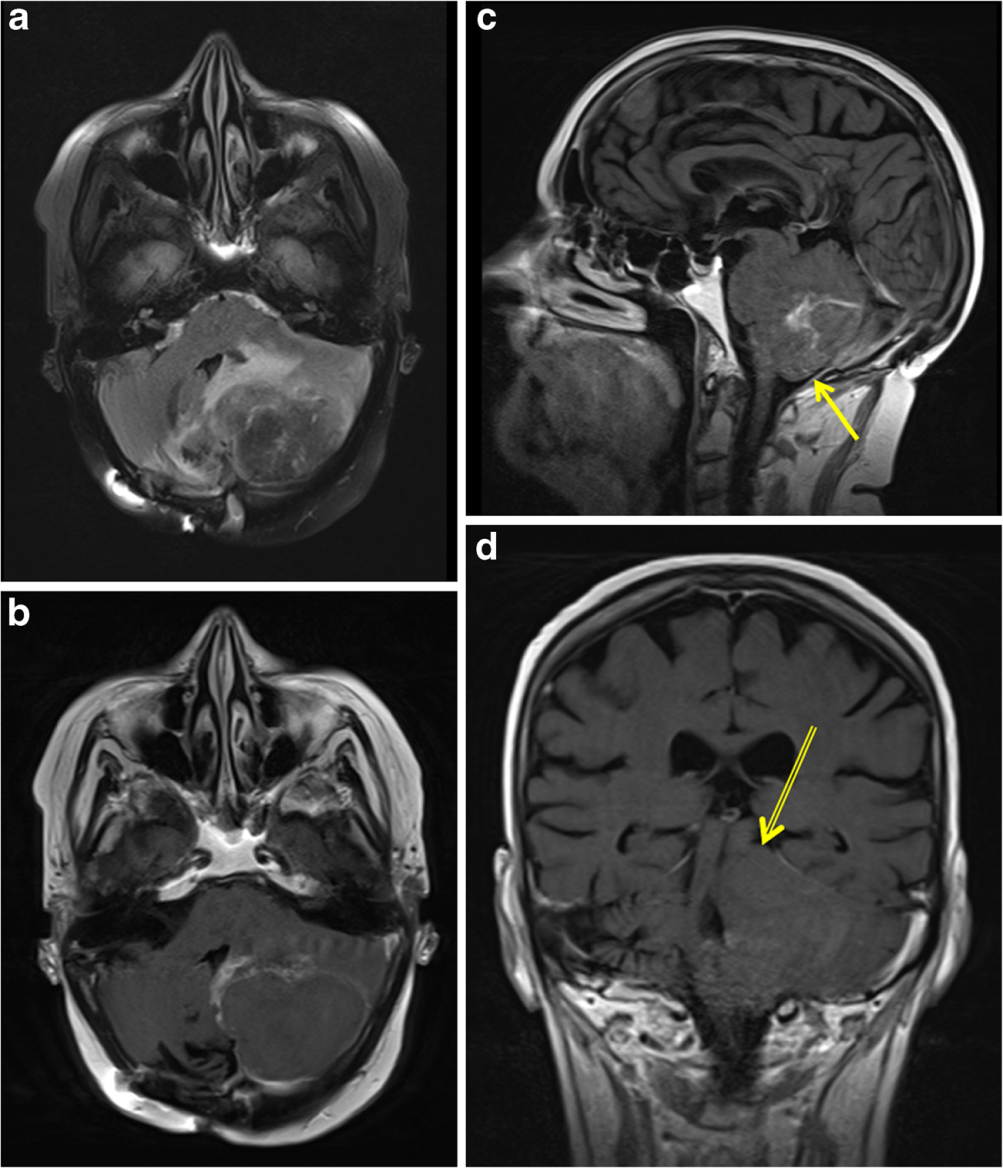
Cerebral herniation secondary to a cerebellar brain metastasis in a 51-year-old woman non-small lung cancer patient with a history of pancranial radiation therapy for brain metastases. Axial FLAIR (a) and axial T1-weighted image after intravenous gadolinium contrast administration (b) show a peripheral enhancing mass in the left cerebellar hemisphere with peritumoural oedema resulting in a midline shift and compression of the fourth ventricle. Sagittal T1-weighted contrast-enhanced image (c) demonstrates herniation of the left cerebellar tonsil trough the foramen magnum (*yellow arrow*). Coronal T1-weighted contrast-enhanced images shows an ascending transtentorial herniation with displacement of cerebellar tissue through the tentorial notch (*double yellow arrow*)
